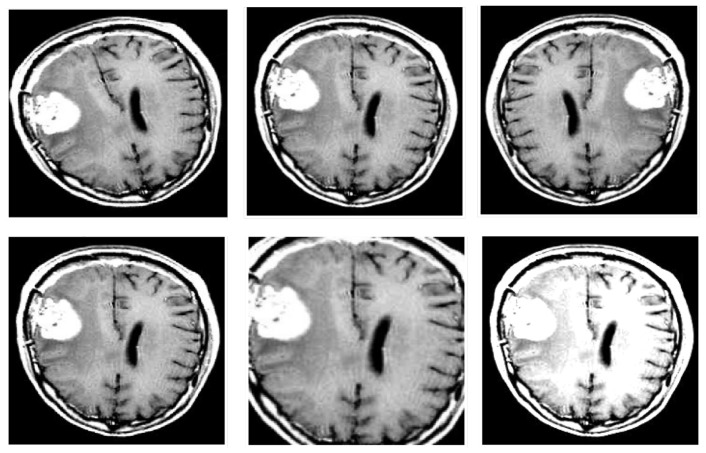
Data augmentation examples on tumor MRI scans, including rotation, flip, brightness shifts, and zoom.
